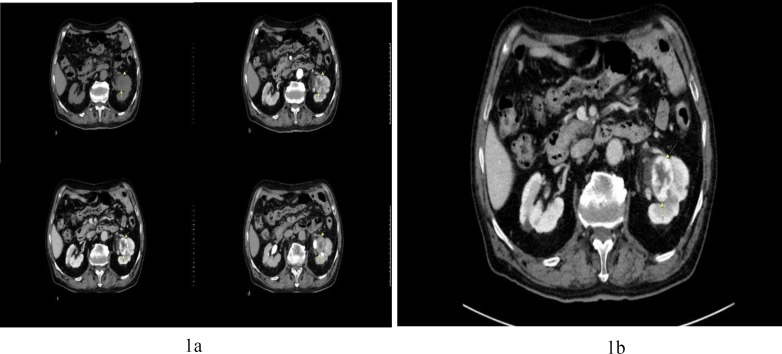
(a) Non-contrast CT scan shows an iso-dense mass. Arterial and portal phase contrast enhanced CT imaging show peripheral nodular enhancement of the lesion with a gradual centripetal fill-in pattern in delayed phase. (b) A solid parapelvic mass with peripheral irregular enhancement in axial contrast CT scan
